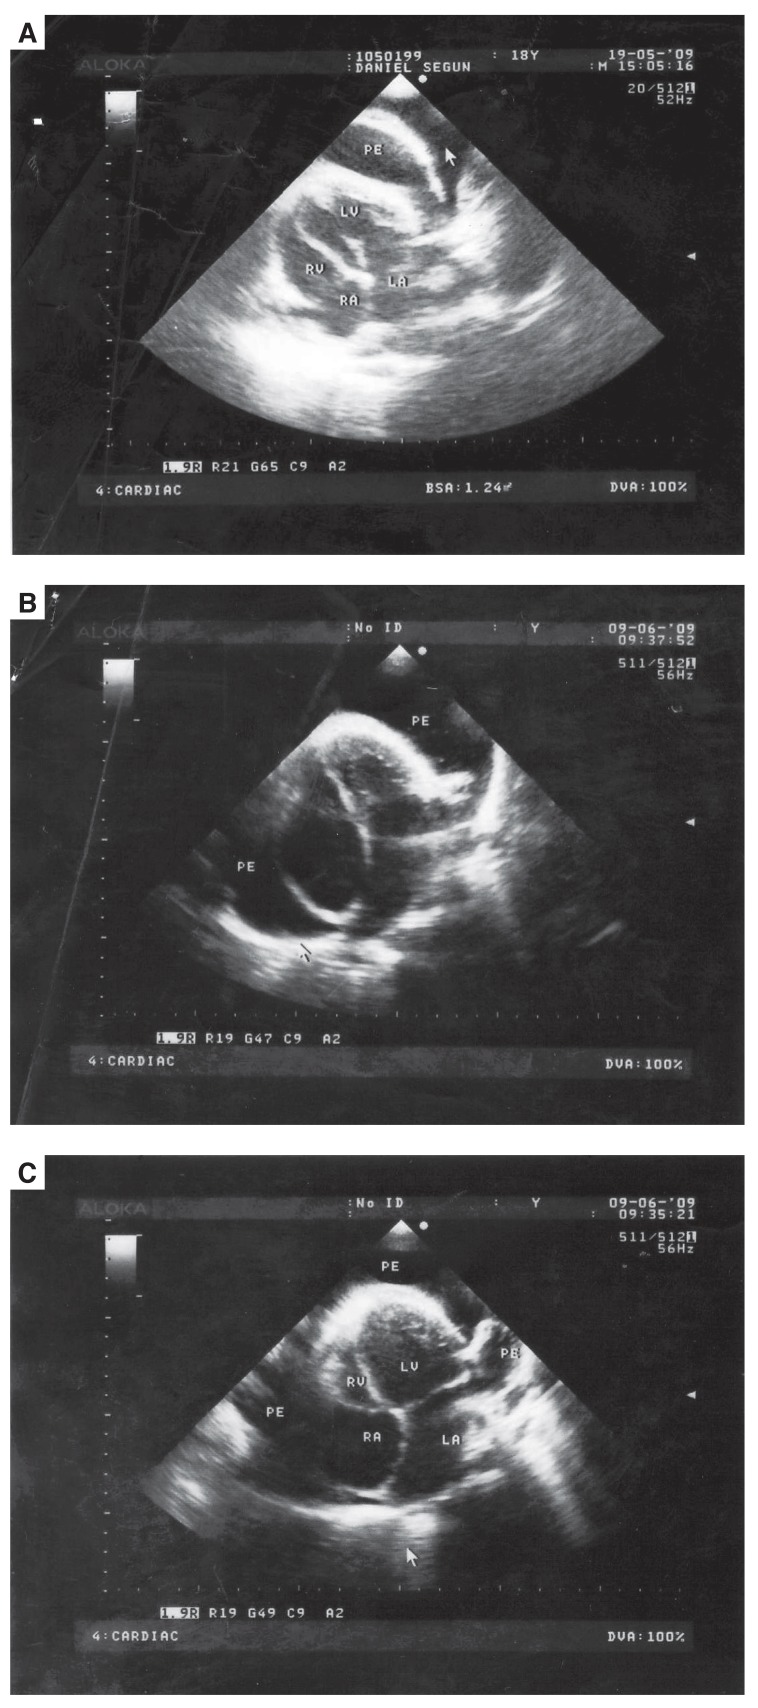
Echocardiography showing moderate pericardial effusion (PE). RV = right ventricle; LV = left ventricle; RA = right atrium; LA = left atrium.
